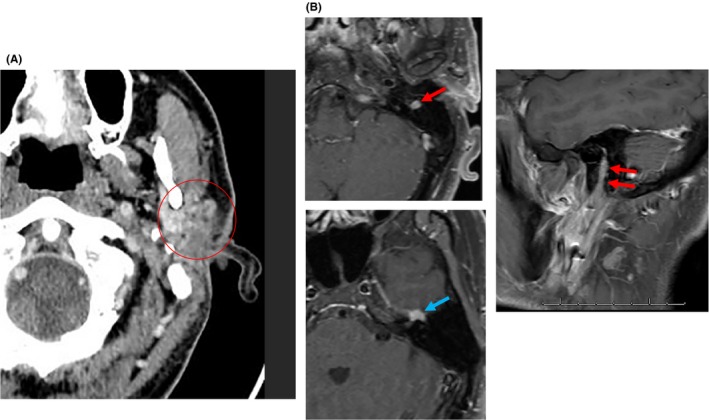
Postoperative imaging. A, Axial postcontrast CT image demonstrating nonspecific postoperative changes in the left parotid bed (red circle). B, Left (axial) and sagittal (right) postcontrast fat‐suppressed T1‐weighted MR images demonstrating enhancing perineural tumor spread along the descending or mastoid facial nerve segment (red arrows) extending to the geniculate ganglion (blue arrow)
